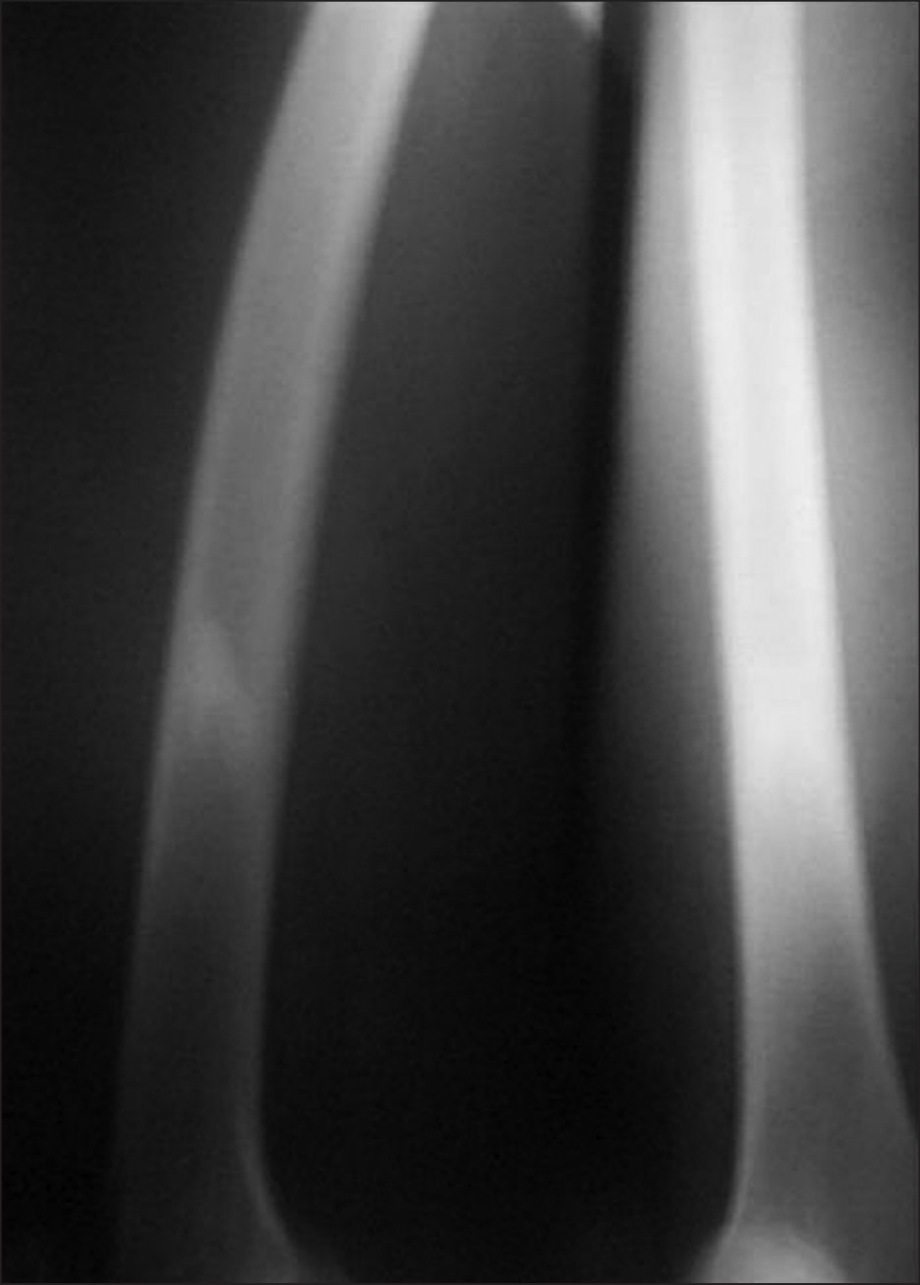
X-ray of the thigh showing only sclerosis
